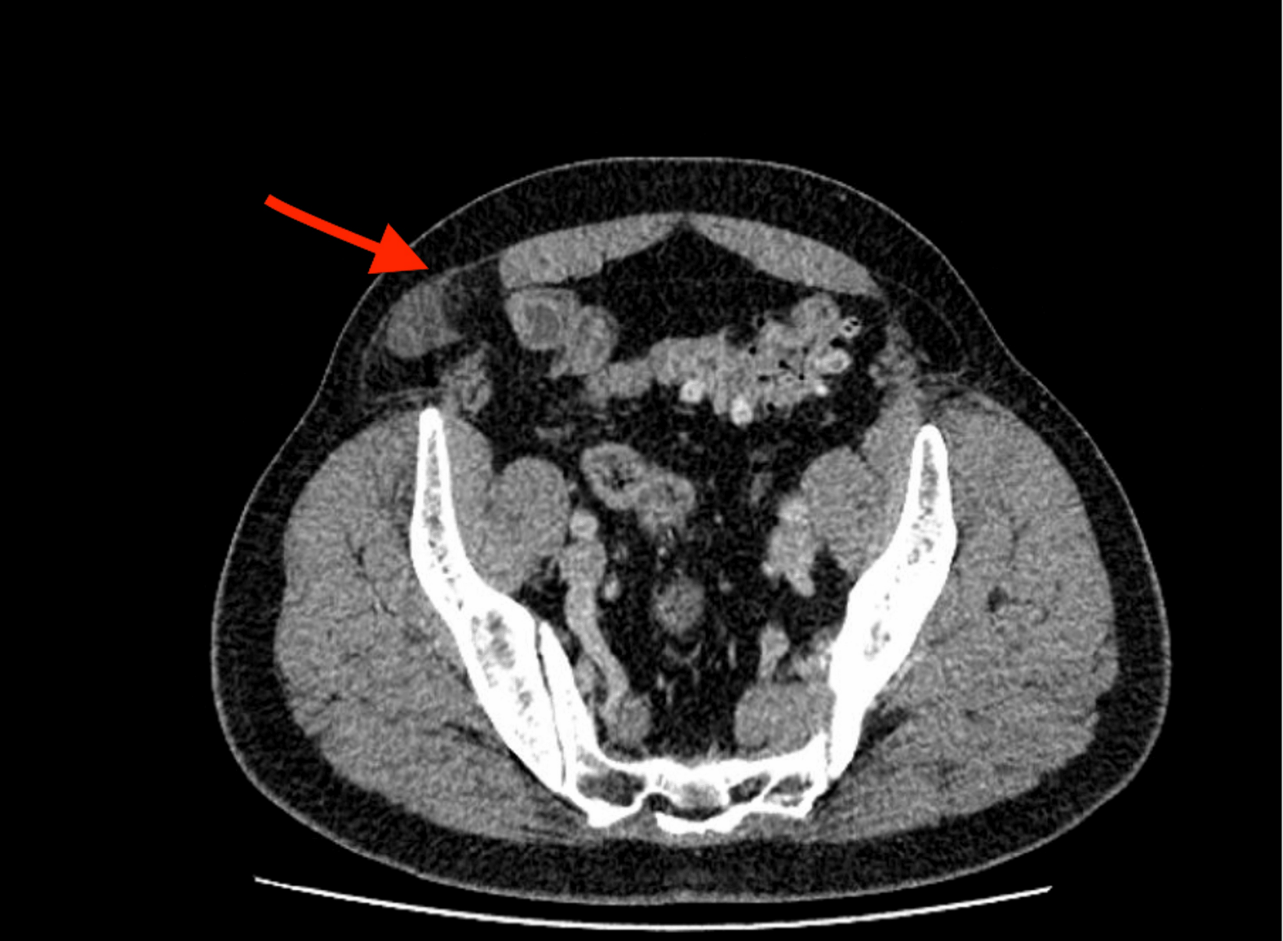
Axial CT scan image showing a Spigelian hernia (red arrow) at the right lateral abdominal wall. A loop of small bowel is seen protruding through a defect in the Spigelian fascia (semilunar line), with adjacent mesenteric fat. Such imaging confirms the presence of an incarcerated Spigelian hernia and often demonstrates any resulting bowel obstruction.
